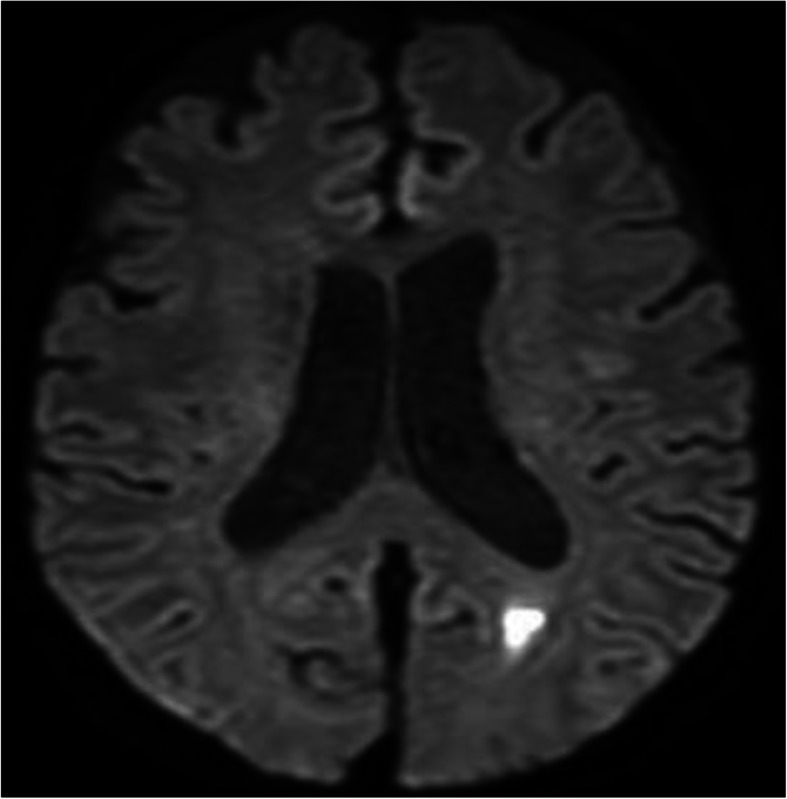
Diffusion-weighted magnetic resonance imaging of the head reveals a high-intensity signal in the left occipital-temporal lobe, which indicates acute cerebral infarction
